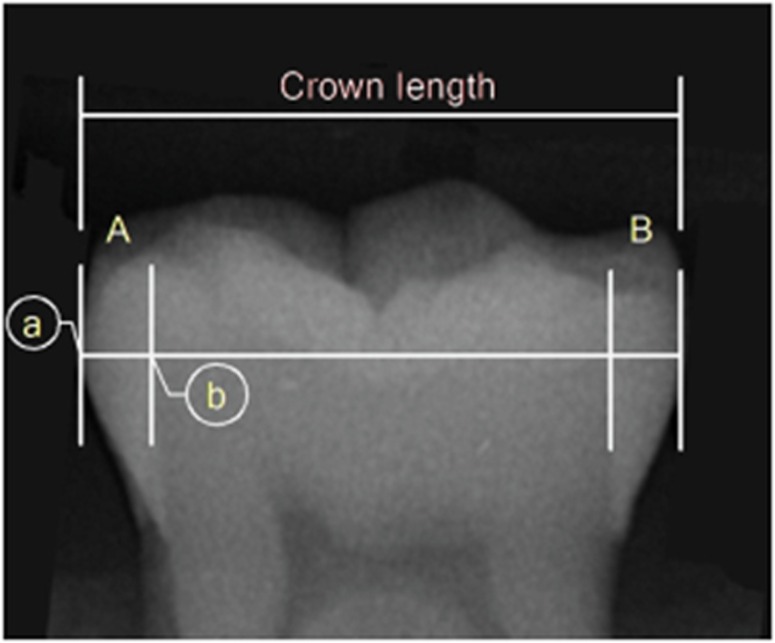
Radiographic tooth measurements. Crown length is the distance from the mesial height of contour to the distal height of contour. A and B are the mesial and distal enamel thickness measured from a to b; a=height of contour, b=the point where the crown length crosses the dentino-enamel junction.
